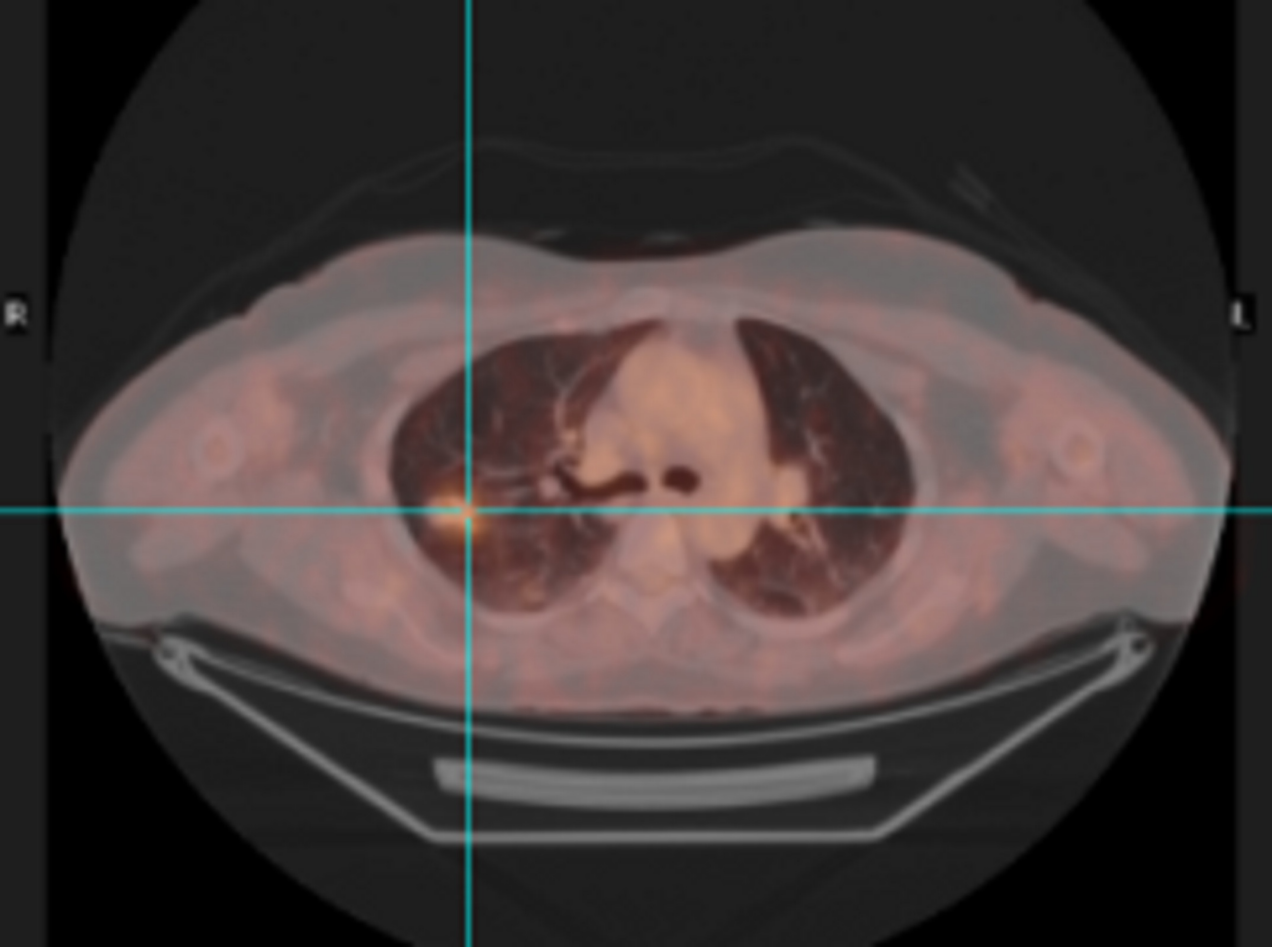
PET/CT scan in March 2013 An FDG-avid right upper lobe nodule with a maximum SUV of 5.2.
